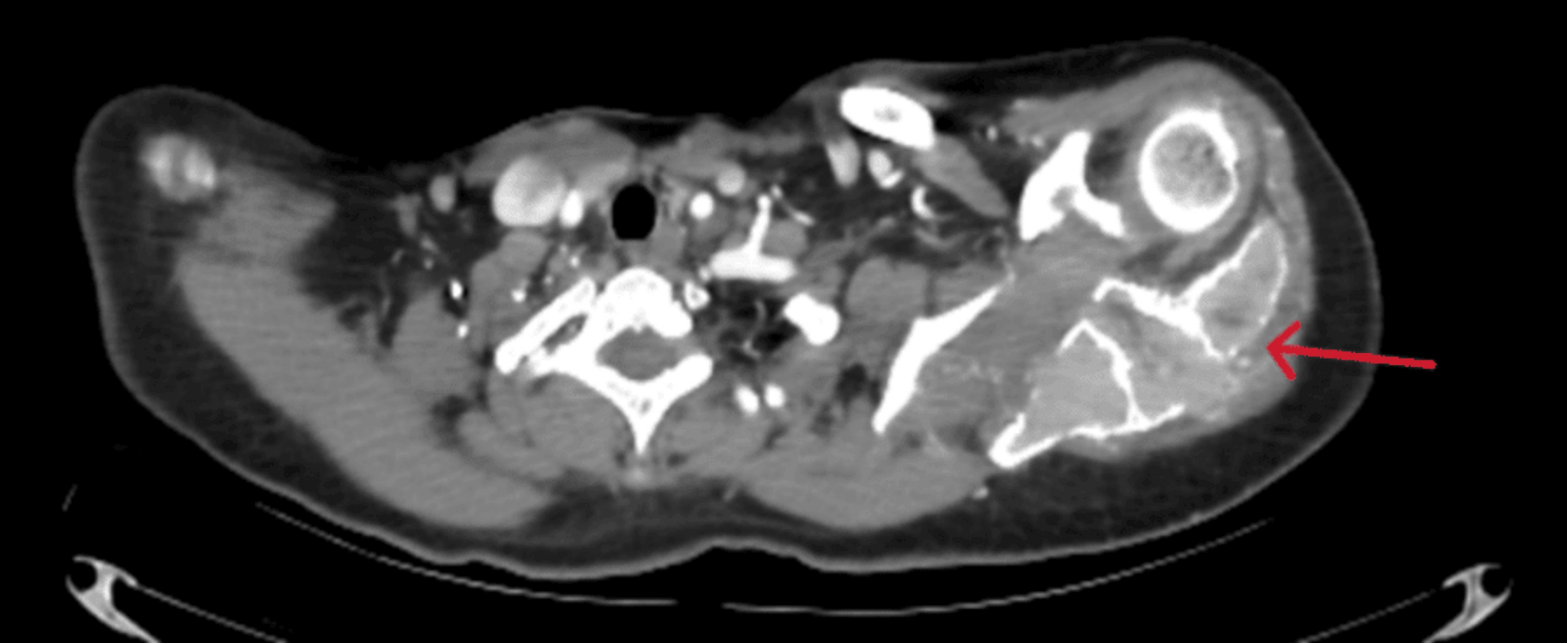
Axial CT scan of the shoulder showing a lesion with irregular contours and cortical bone disruption, extending into the adjacent soft tissues near the deltoid muscle (arrow).
